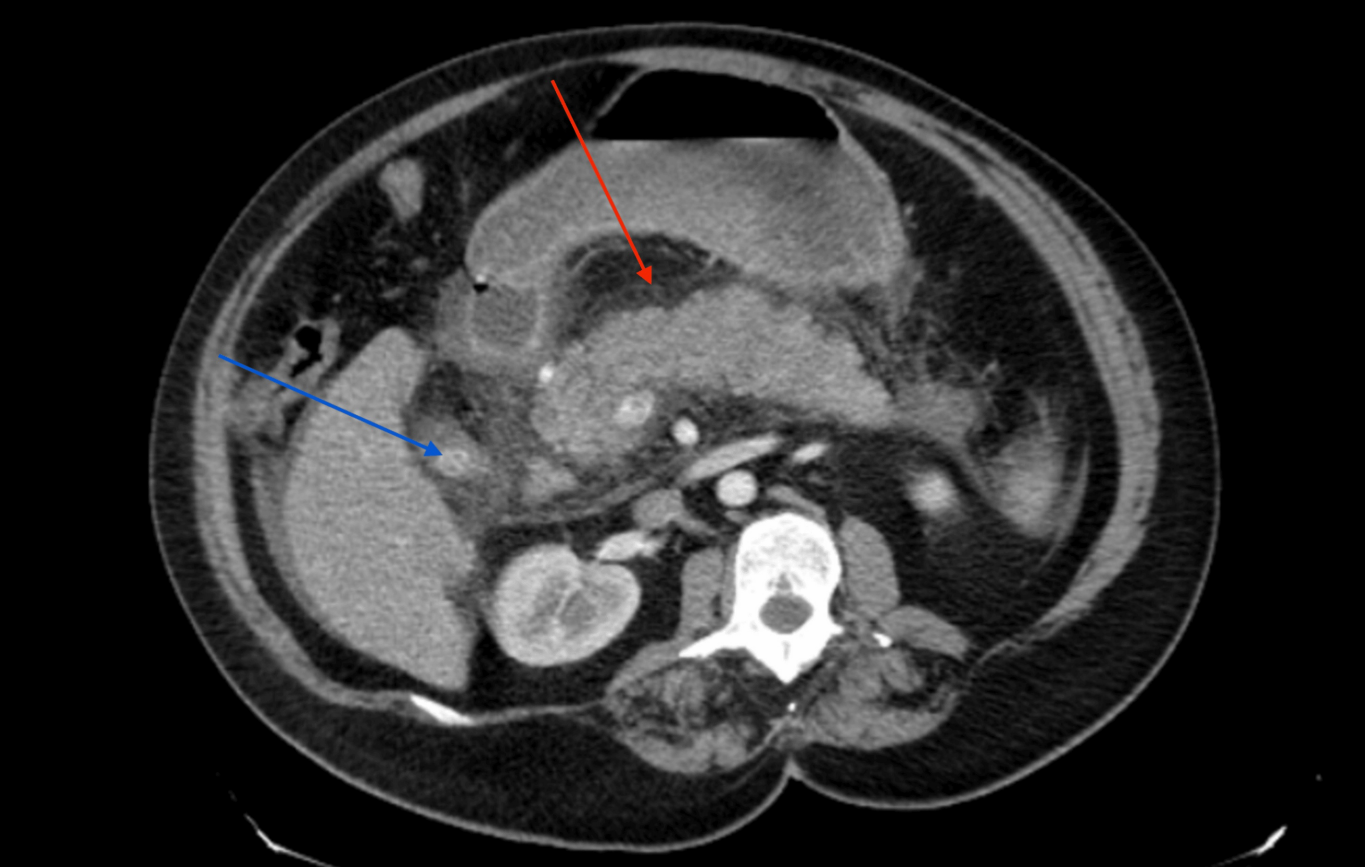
CT scan of the abdomen and pelvis The red arrow indicates acute pancreatitis with peri-pancreatic edema and linear fluid, and the blue arrow indicates a 1.3 cm gallstone.
